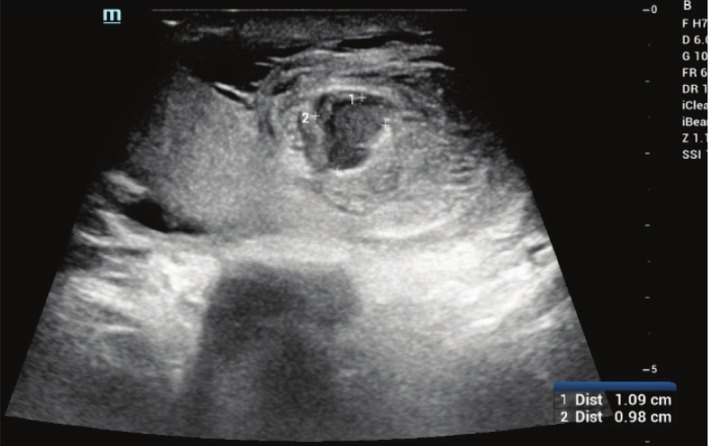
Ultrasound shows scrotal ultrasound demonstrates a normal right testicle (arrow) with preserved echotexture.
